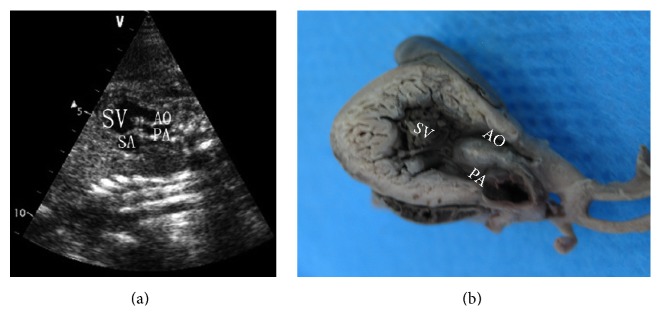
Single ventricle (SV) (24 w). (a) The long axis of cardiac apex echocardiography section. (b) The long axis of cardiac apex sectional anatomy. SV: single ventricle; AO: aorta; PA: pulmonary artery; SA: single atrium.
